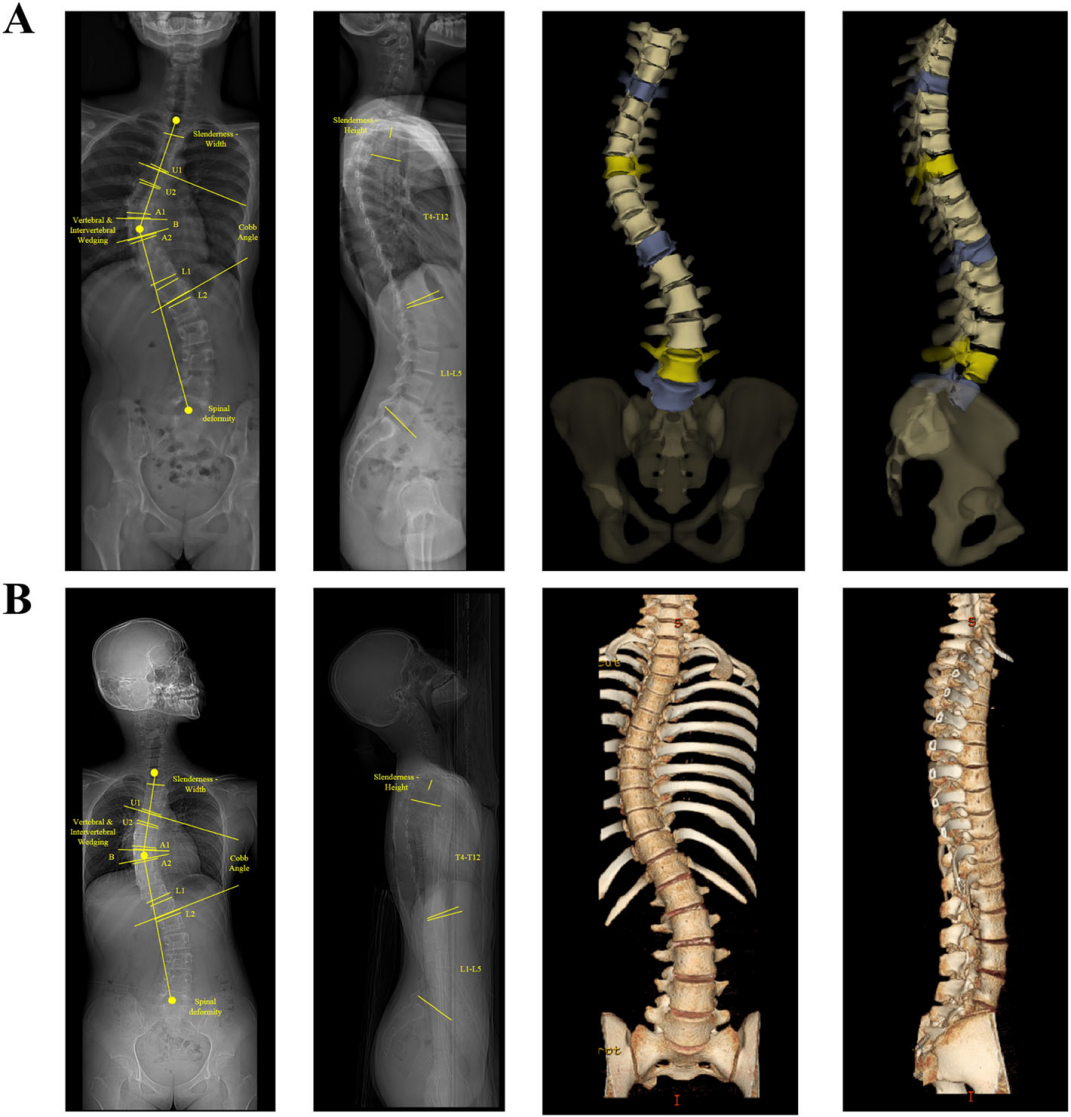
The radiographs of a 16-year-old female AIS patient with the frontal and lateral views, and 3D reconstruction images (a – EOS bi-planar stereoradiography; b – CT digital reconstructed radiography)
